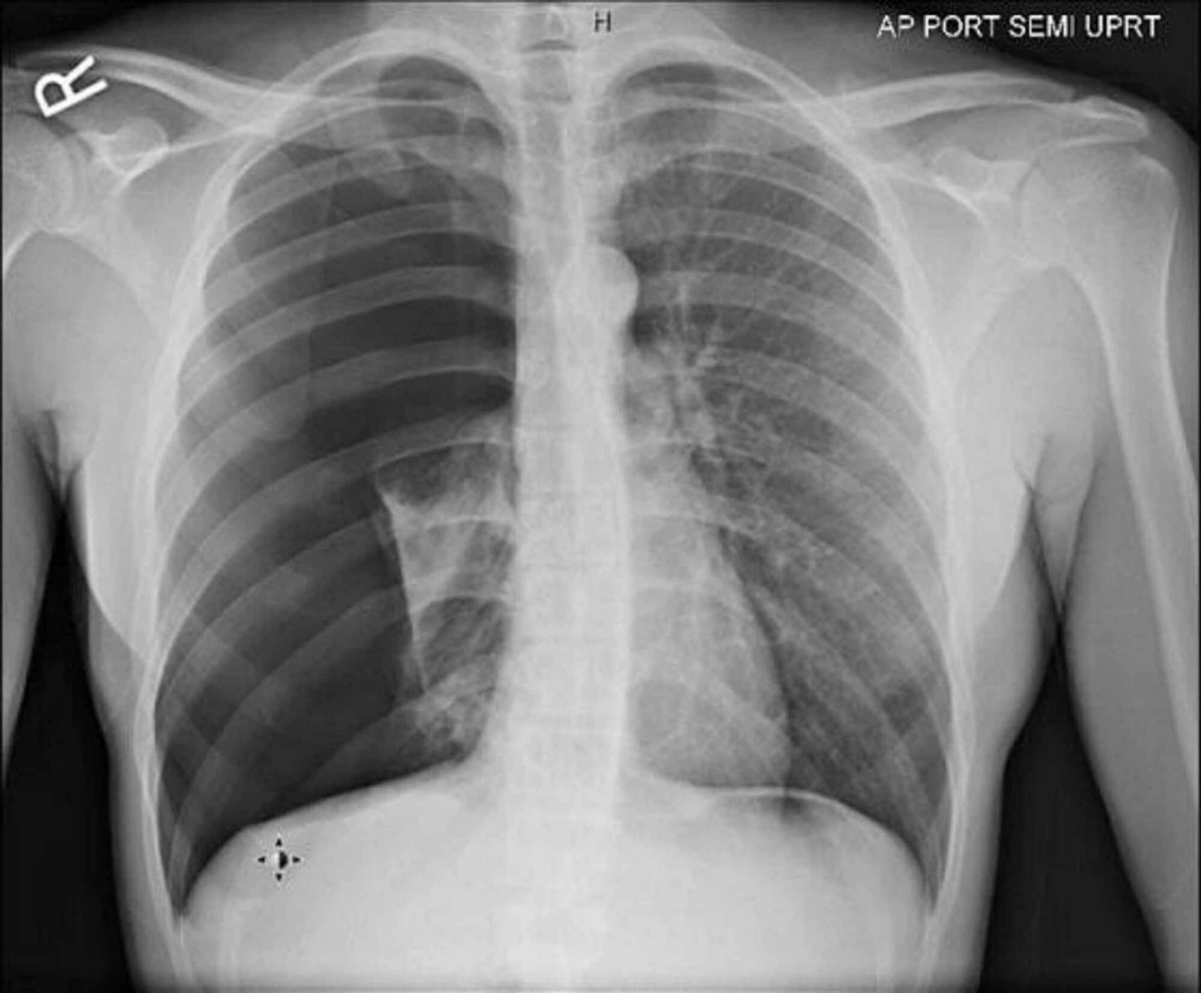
Chest x-ray (CXR) shows large right-sided pneumothorax with a complete collapse of the right lung.
